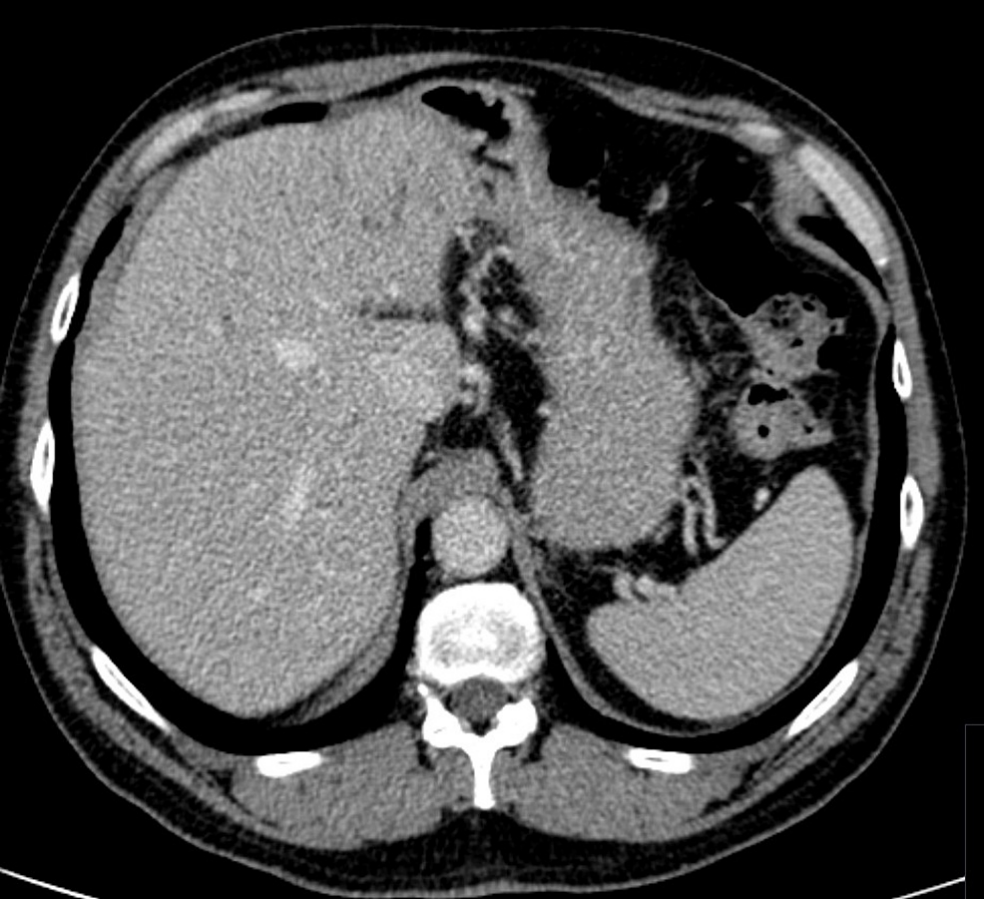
Normal CT scan of the abdomen.
